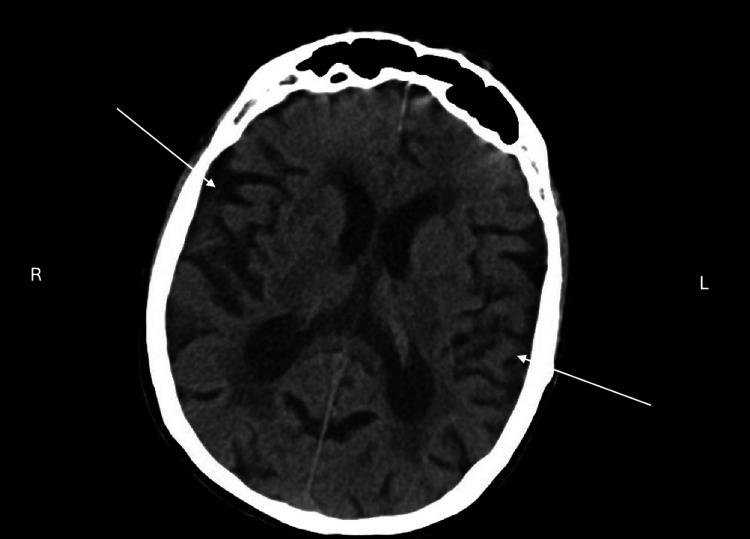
CT brain without contrast, transverse view. Diffuse parenchymal volume loss, more pronounced in the right greater than left bilateral anterior mesial temporal lobes.
